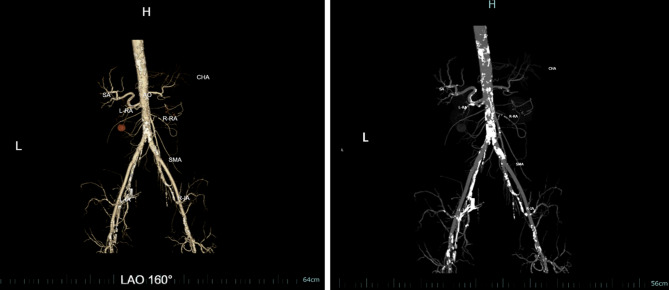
Abdominal vascular CTA showing extensive atherosclerotic lesions, arterial stenosis and penetrating ulcer of the abdominal aorta and its branches
